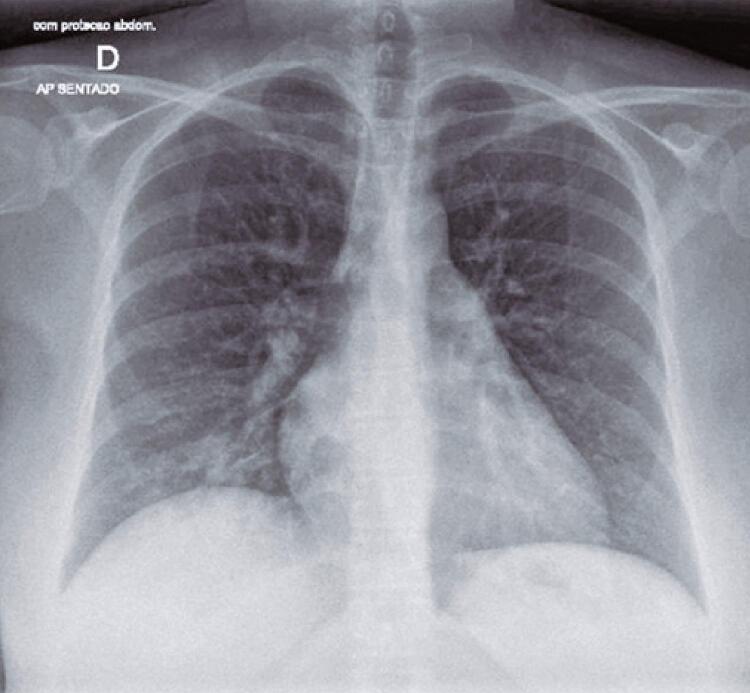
Anteroposterior chest X-ray with interstitial involvement and mild opacity at the right lung base
